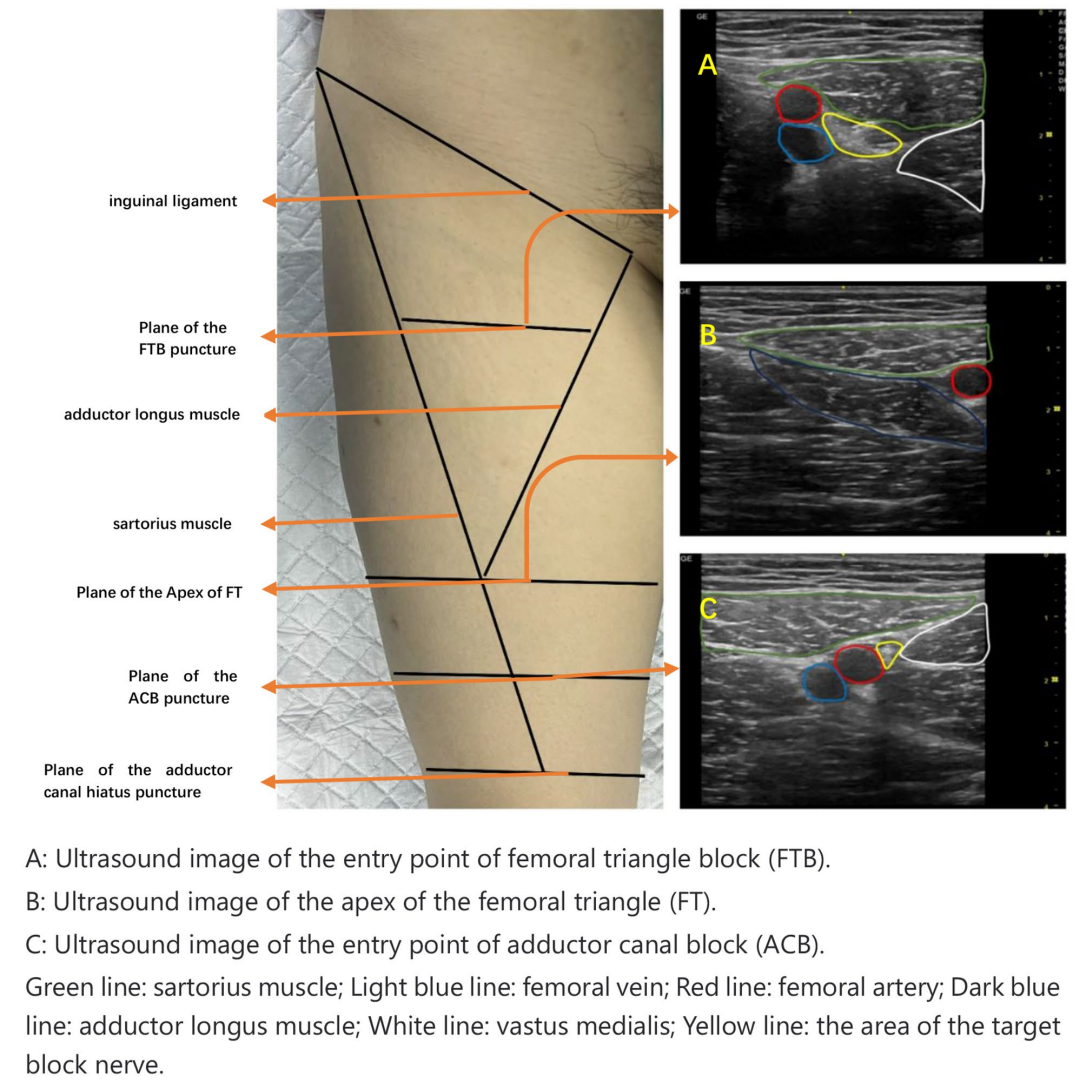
Ultrasound image of the plane of the puncture site
